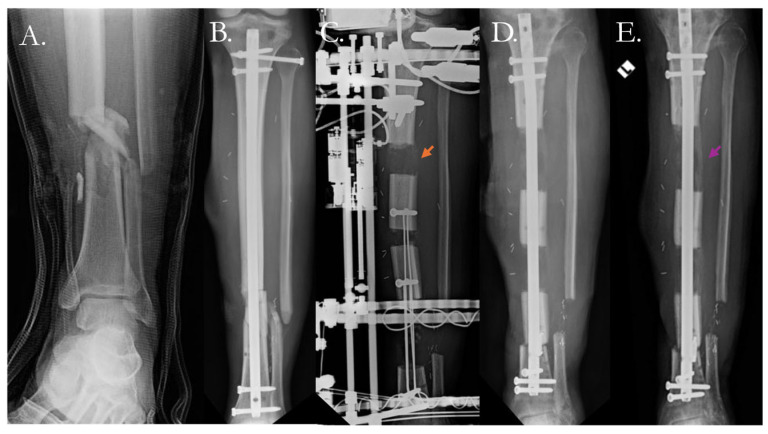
Distraction osteogenesis of the tibia following nonunion. 57-year-old male with an open tibial and fibular septic nonunion following a motorcycle collision. (A) Radiograph of initial injury demonstrating a displaced segmental distal third tibia fracture with ipsilateral fibula fracture. (B) Initial fracture fixation using intramedullary nailing to allow for secondary bone healing. (C) Radiograph after debridement and saucerization of regions of tibial osteomyelitis with dynamic external fixator in place. Region of regenerate highlighted with orange arrow. (D) Definitive fixation with intramedullary nail after removal of dynamic external fixator at 4 months after initial external fixator placement. (E) Most recent radiograph at 6 weeks after intramedullary nail placement with evidence of early callus about region of regenerate (purple arrow).
